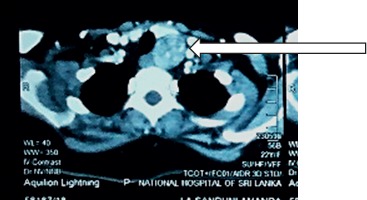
CT neck showing the left side parathyroid mass.
